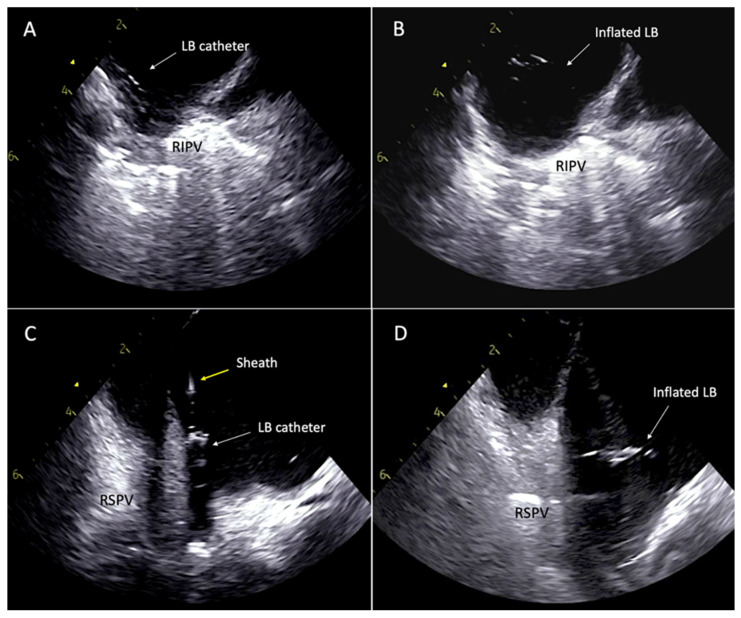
LB: laser balloon; RIPV: right inferior pulmonary vein; RSPV: right superior pulmonary vein; ICE: Intracardiac echocardiography. Low fluoroscopy ICE-guided approach for LB ablation. (A,B) LB catheter directed into RIPV using ICE guidance and inflated prior to ablation. (C,D) RSPV cannulated under ICE and inflated into antral position.
